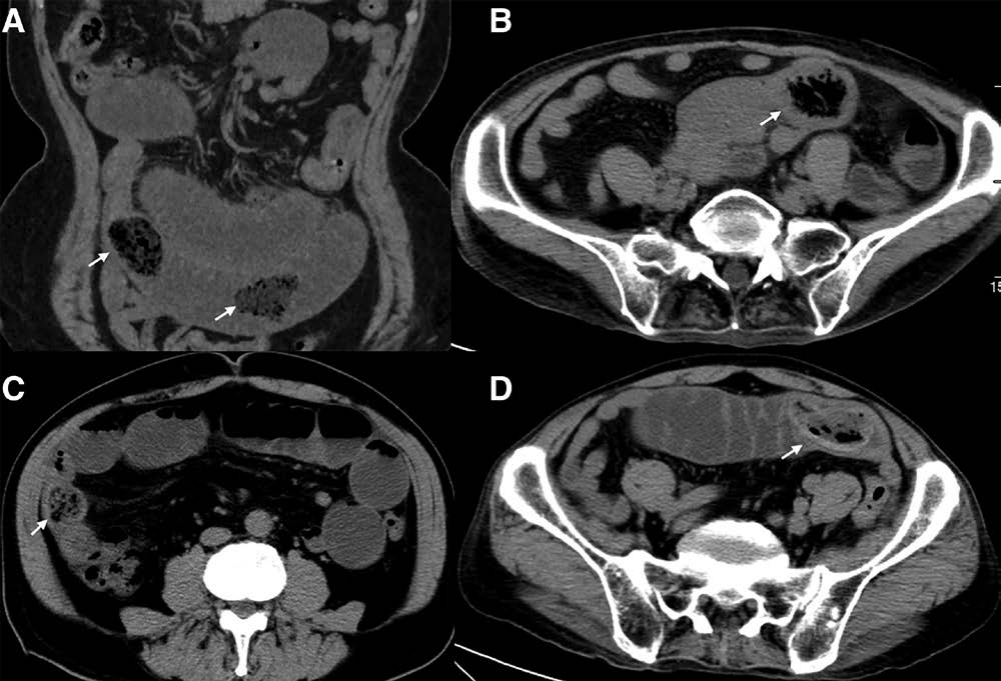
CT scans in the coronal and axial planes show five primary enteroliths that appear to be unevenly low-density with a mottled gas appearance (arrow). (A) Two stones of a 67-year-old-femal in the jejunum, one appears oval and the other irregular. (B) A round-like stone of a 72-year-old-femal located in the jejunum. (C) A round-like stone of a 74-year-old-man at the junction of jejunum and ileum. (D) An oval stone of a 78-year-old-man located in the jejunum with a slightly higher density ring. CT = computed tomography.
